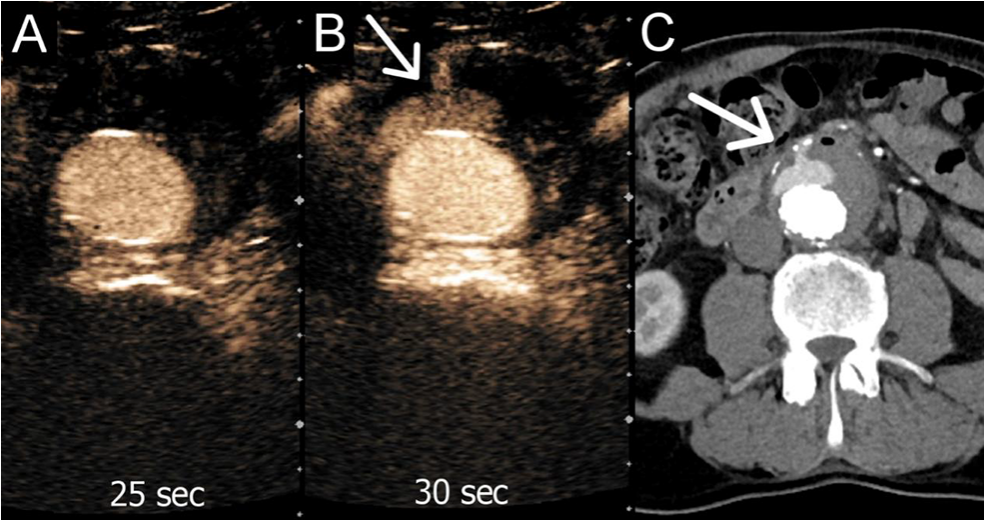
Fig. 1 Type II endoleak. The endoleak (b) occurs with delay over contrast filling of the stengraft (a). Endoleak is indicated by the arrow in image b. CT image correlates with US image (c), endoleak is highlighted by the arrow
